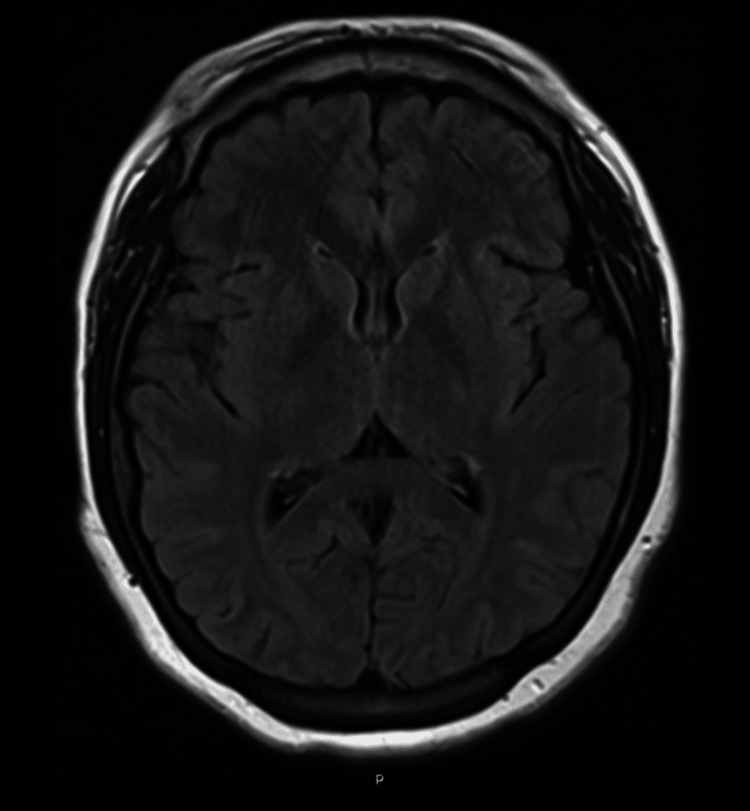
Axial FLAIR MRI with normal-appearing cortical and subcortical brain parenchyma as well as normal-appearing ventricles. FLAIR MRI: Fluid-attenuated inversion recovery magnetic resonance imaging
